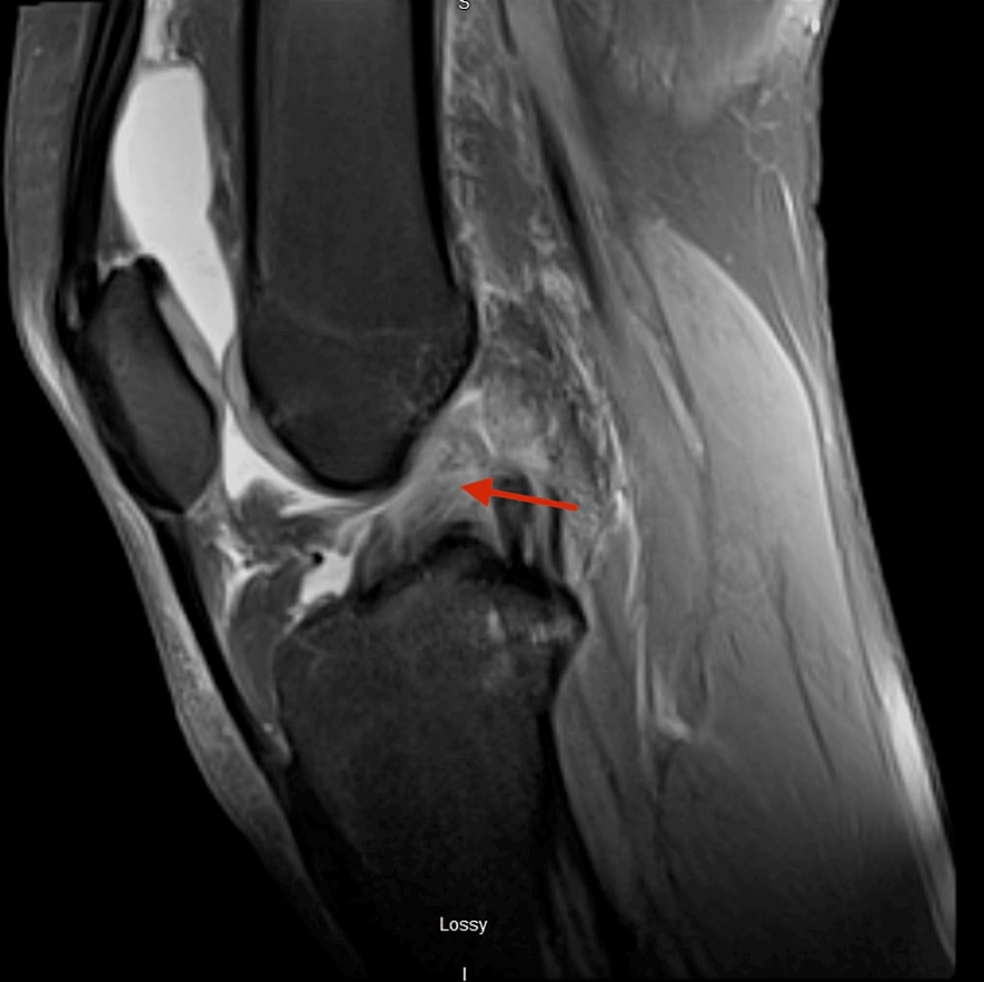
T2 sagittal magnetic resonance imaging of the left knee The image shows the preoperative anterior cruciate rupture (red arrow).
